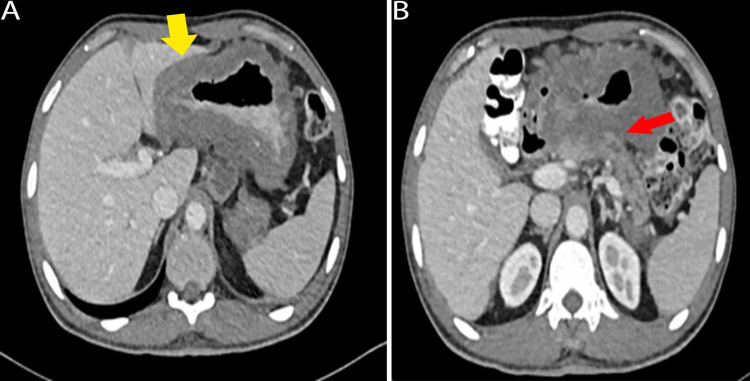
Axial CT of the chest, abdomen, and pelvis with oral and IV contrast (A, B) A) Diffuse thickening of the gastric wall, especially at the lesser curvature, is noted, appearing inseparable from the adjacent hepatic parenchyma (yellow arrow); B) The mass extends to infiltrate the adjacent pancreatic parenchyma (red arrow), with multiple perigastric lymph nodes and numerous omental and peritoneal nodules
